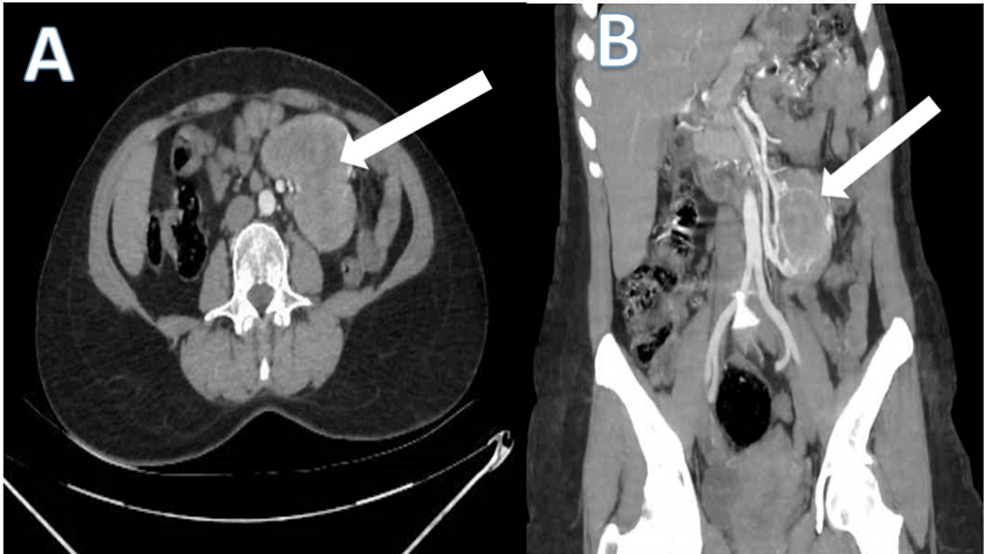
Abdominal CT showing a mesenteric mass with intense and heterogeneous contrast enhancement (white arrow) (A) Sectional axial image. (B) Coronal section.
